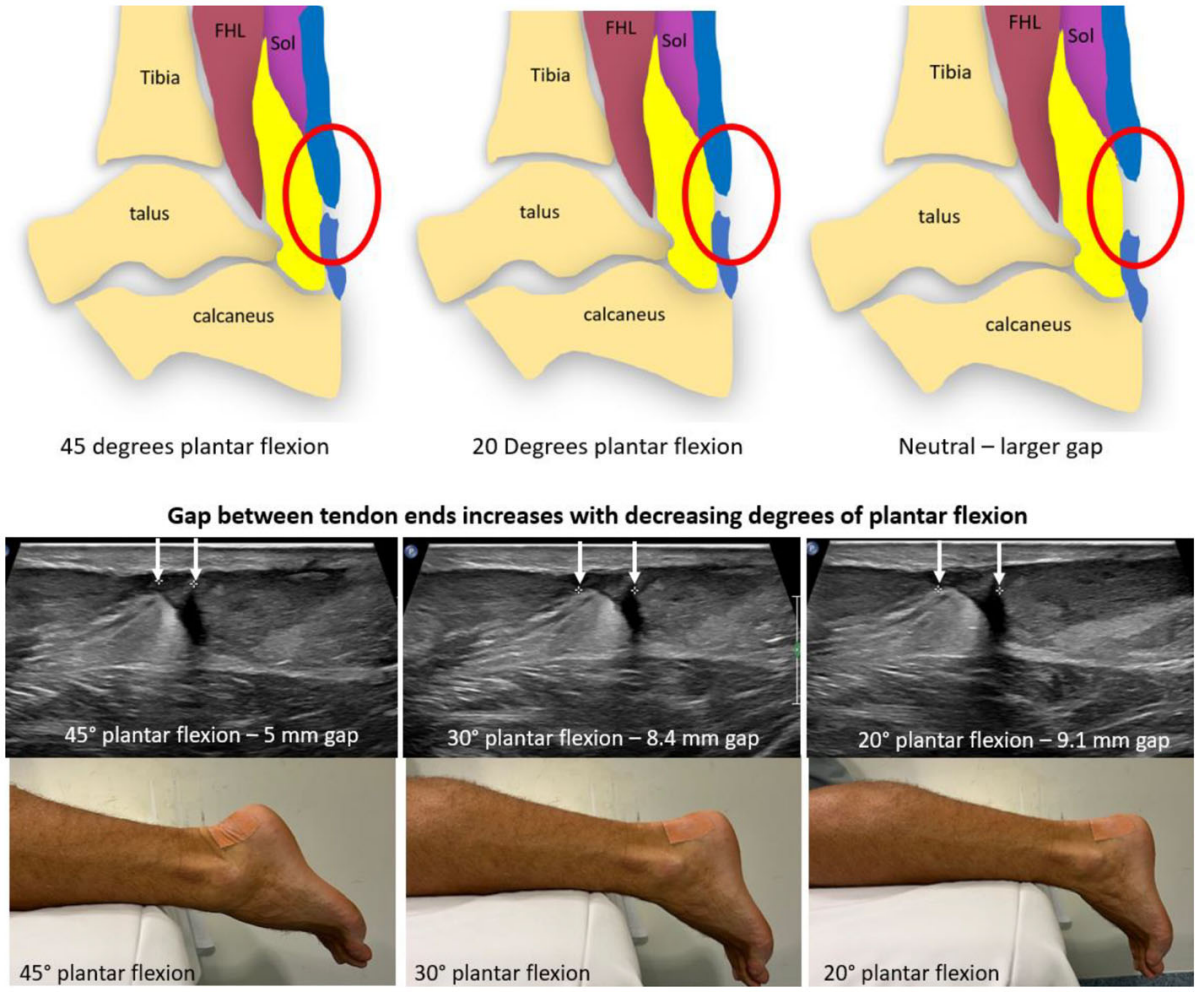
(a) Position of torn Achilles tendon ends (blue) with varying degrees of plantar flexion. As plantar flexion is reduced, the gap (outlined by red oval) between tendon ends becomes greater. (b) Long‐axis sonographic images acquired to measure the gap between ruptured Achilles tendon ends which increased with decreasing degrees of plantar flexion (demonstrated by accompanying photos). FHL, flexor hallucis longus muscle; Sol, soleus muscle.
